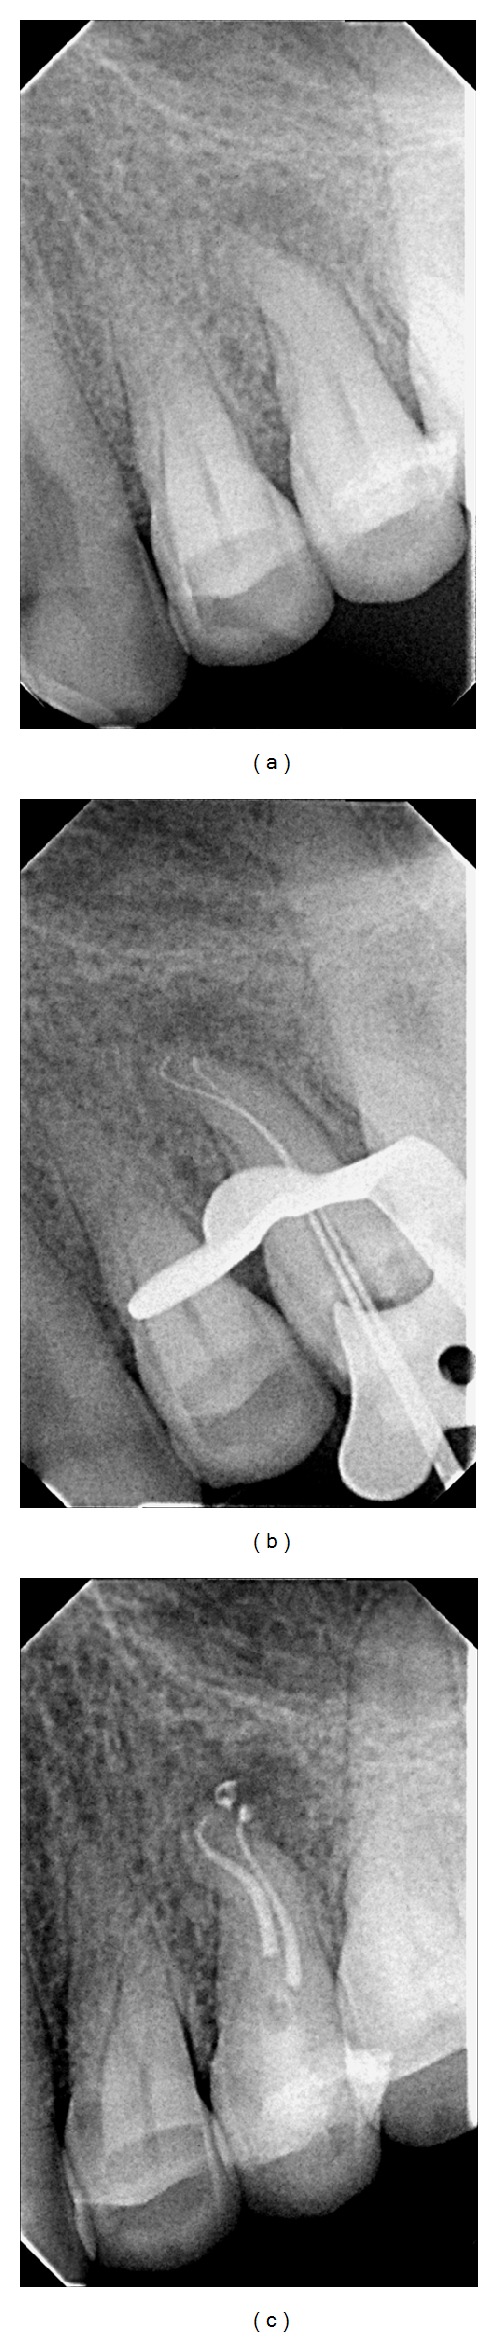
(a) Initial radiograph showing periapical radiolucency, (b) radiographic confirmation of the working length, and (c) immediate posttreatment radiograph.
